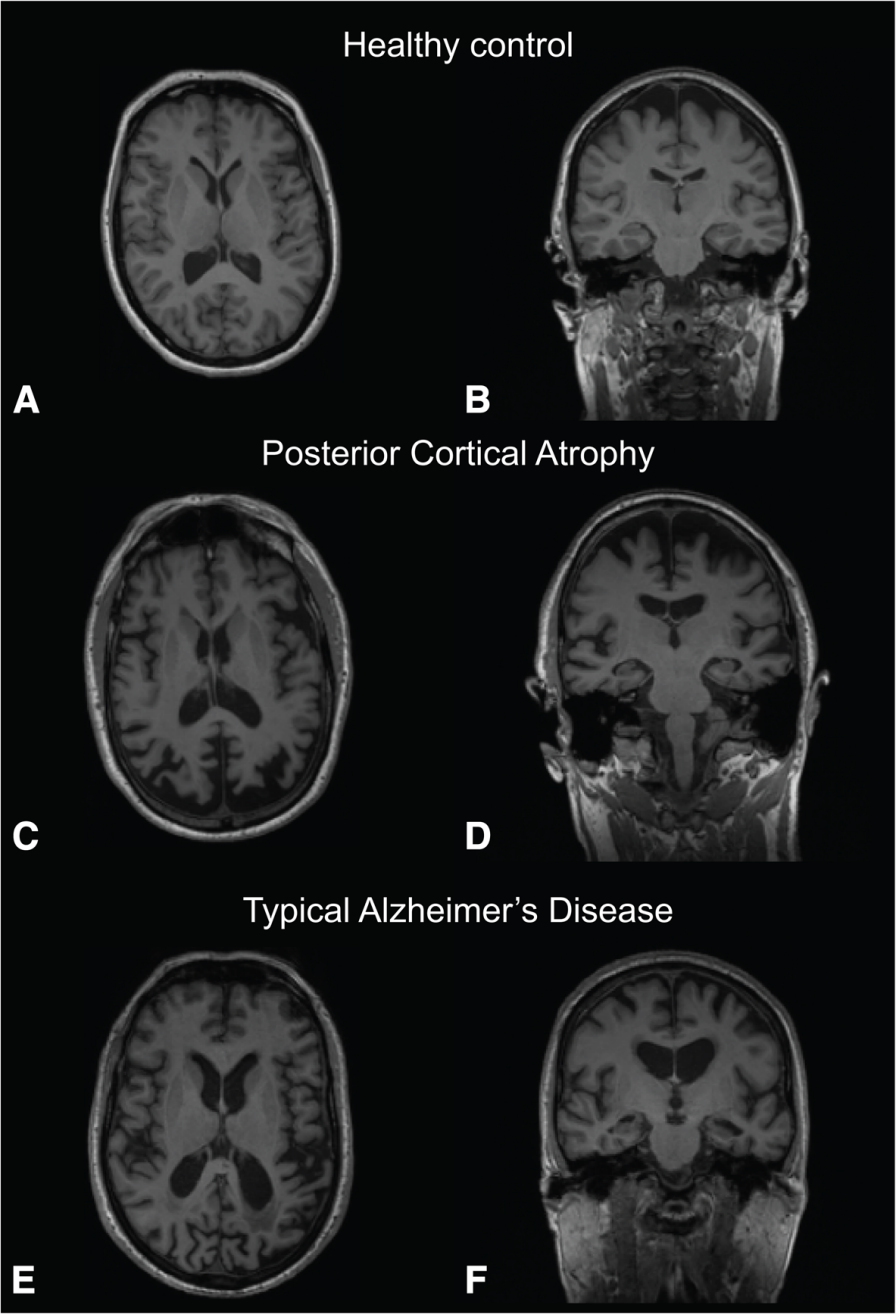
Neurodegenerative features on T1 MRI. Transverse (a, c, e) and coronal (b, d, f) representative T1-weighted volumetric MRI scans in a control, a posterior cortical atrophy (PCA) and a typical Alzheimer’s disease (tAD) case (left hemisphere is shown on the right and vice versa). The PCA case shows predominant parietal-occipital atrophy (c) and relatively preserved hippocampal volume (d) while the tAD case shows evidence of more widespread neocortical atrophy, although with relatively preserved occipital volume (e) and more extensive hippocampal volume loss (f)
